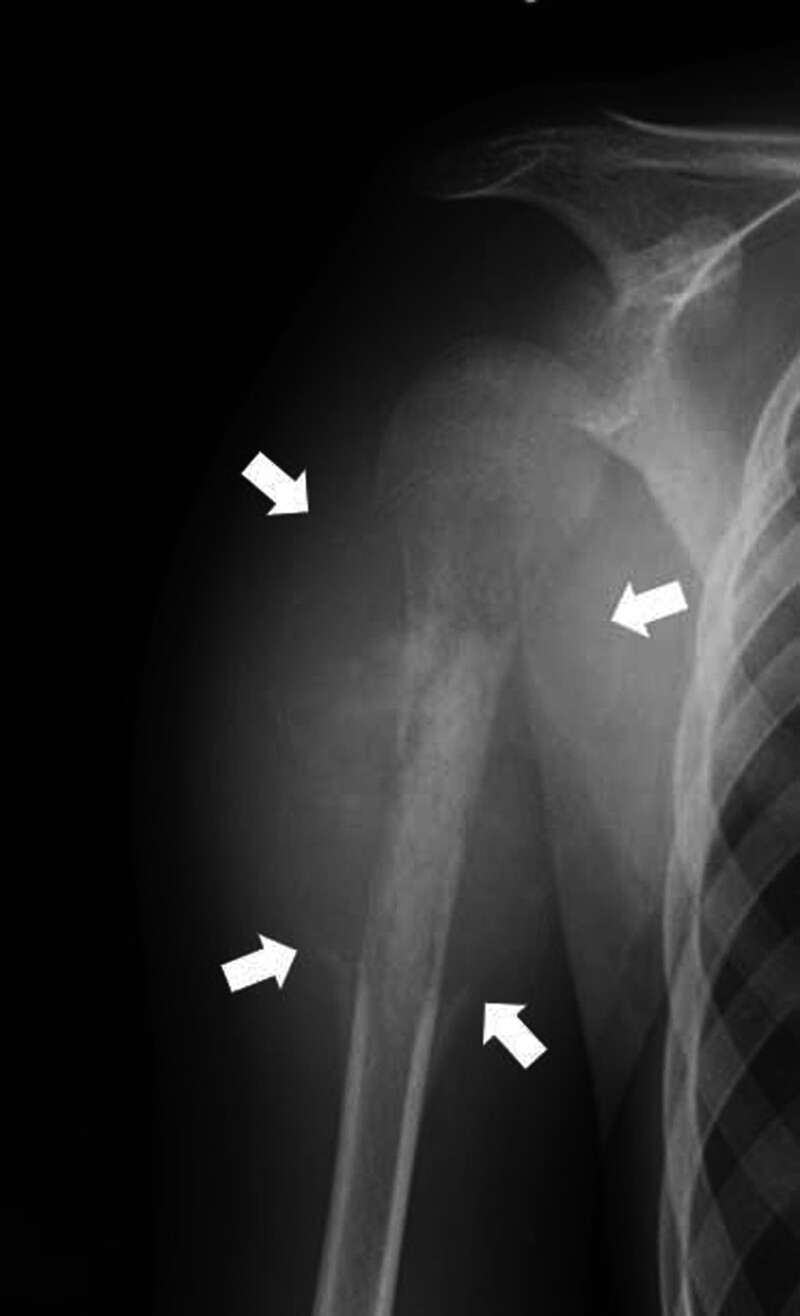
Plain radiograph of a 12-yr-old girl with osteoblastic osteosarcoma in the right proximal humerus. The periosteal reaction of sunburst appearance/spicula/Codman’s triangle, matrix of abnormal osteoblastic change and new bone formation, soft tissue extension, ill-defined border, and permeative-type bone destruction were observed. CT = computed tomography, MRI = magnetic resonance imaging.
